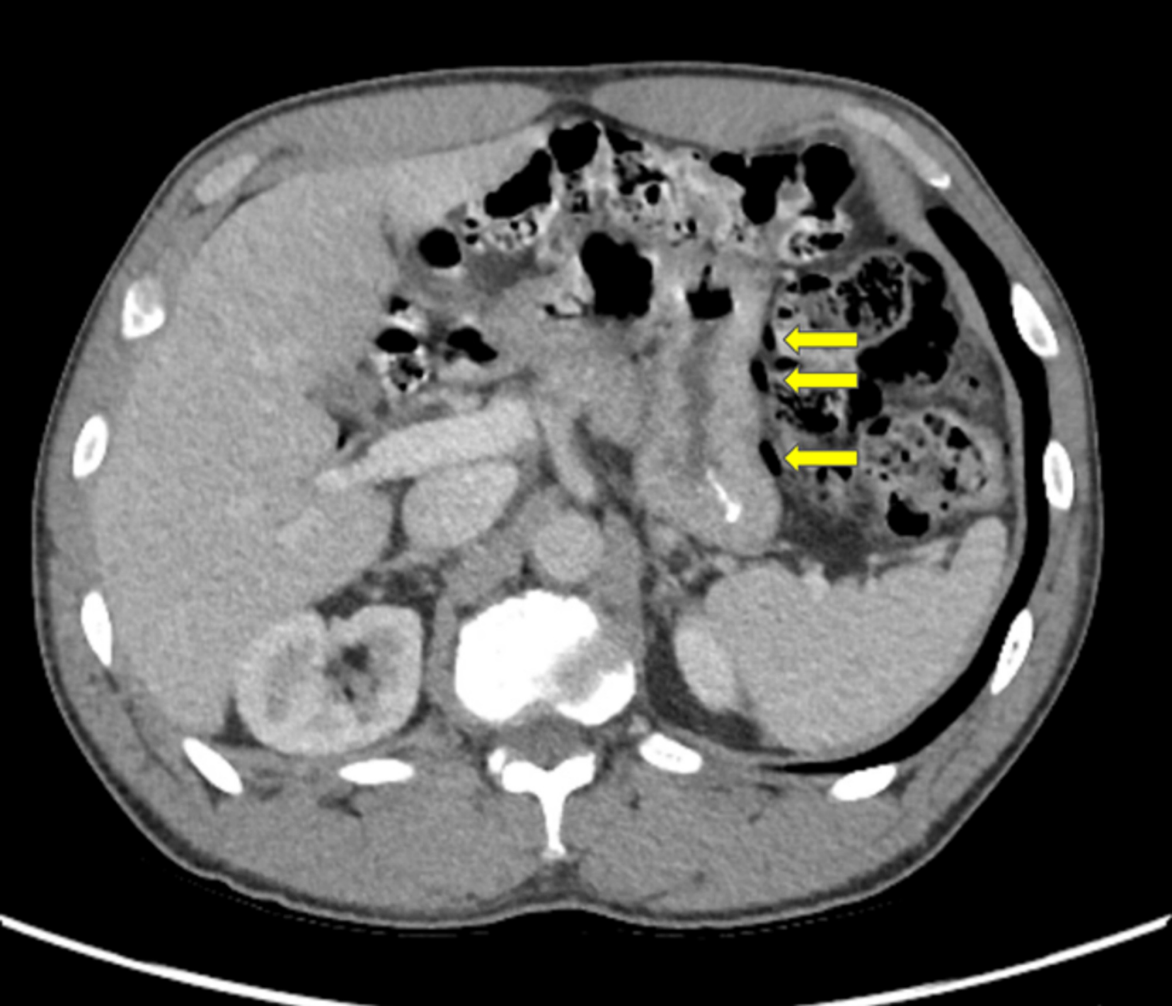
Follow-up CT of the abdomen and pelvis with contrast Computed tomography (CT) with curvilinear foci of air in the wall of the large bowel at the splenic flexure without bowel wall thickening.
